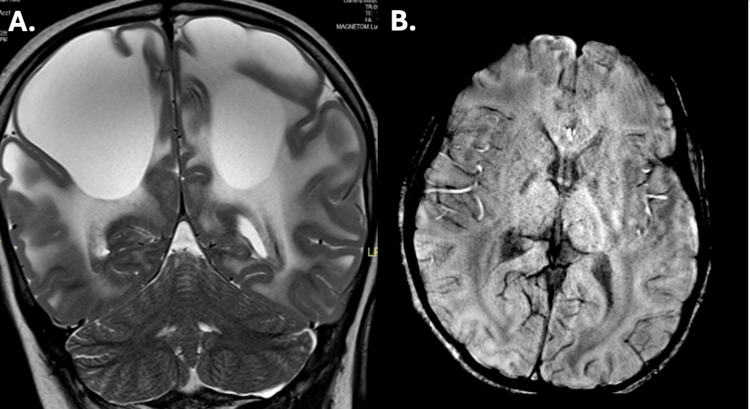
(A) Coronal T2W 3-T MRI image demonstrating the large occipito-parietal cysts with sparing of posterior fossa structures and (B) axial T2*GRE 3-T MRI image showing bilateral normal basal ganglia without calcifications. T2W, T2 weighted; GRE, gradient recalled echo
